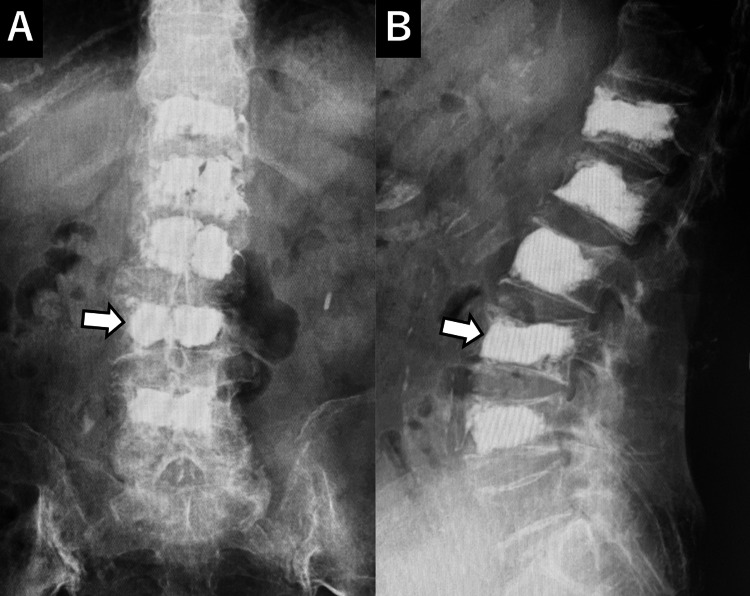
Post-third BKP X-ray An X-ray taken after the third balloon kyphoplasty (BKP) shows bone cement in L3 (arrow).
